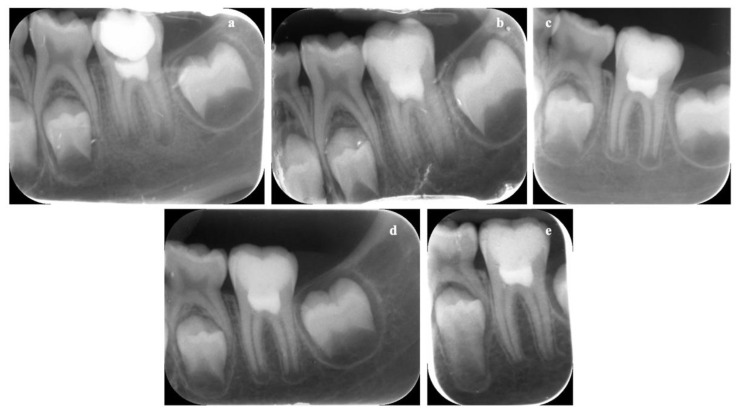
Periapical radiographs of dental element (3.6) treated with ProRoot MTA. The immature permanent tooth is shown immediately after vital pulp therapy (Baseline) (a) and after 1 month (b), 3 months (c), and 1 (d) and 2 years of follow-up (e). Progressive formation of the dental roots could be observed over time. Closure of apical foramina is clearly represented after 2 years of follow-up.
